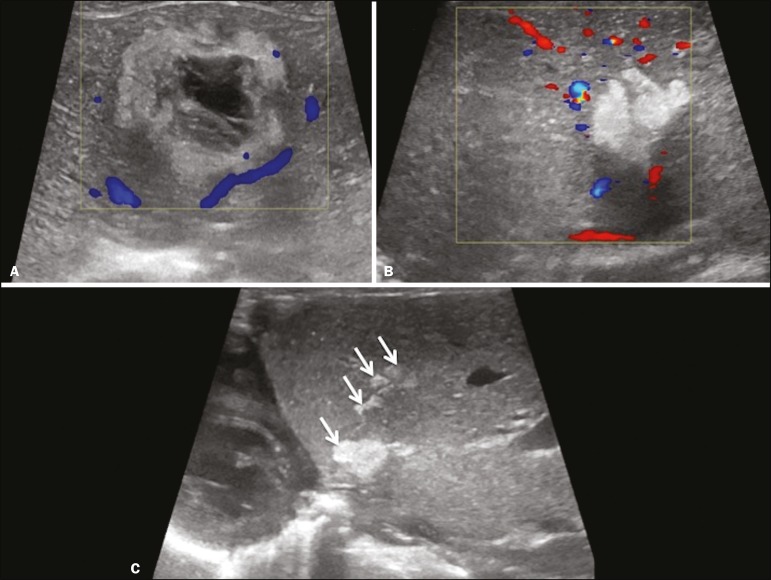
Hematoma as a complication of misplaced umbilical catheter. Ultrasound examinations of three different newborns, at two, four, and six weeks of age, respectively, showing hyperechoic focal lesions with irregular borders. In A, there is a liquid component in the center of the lesion. In C, the lesion is elongated (arrows), probably representing the path of the catheter that transfixed the hepatic parenchyma.
